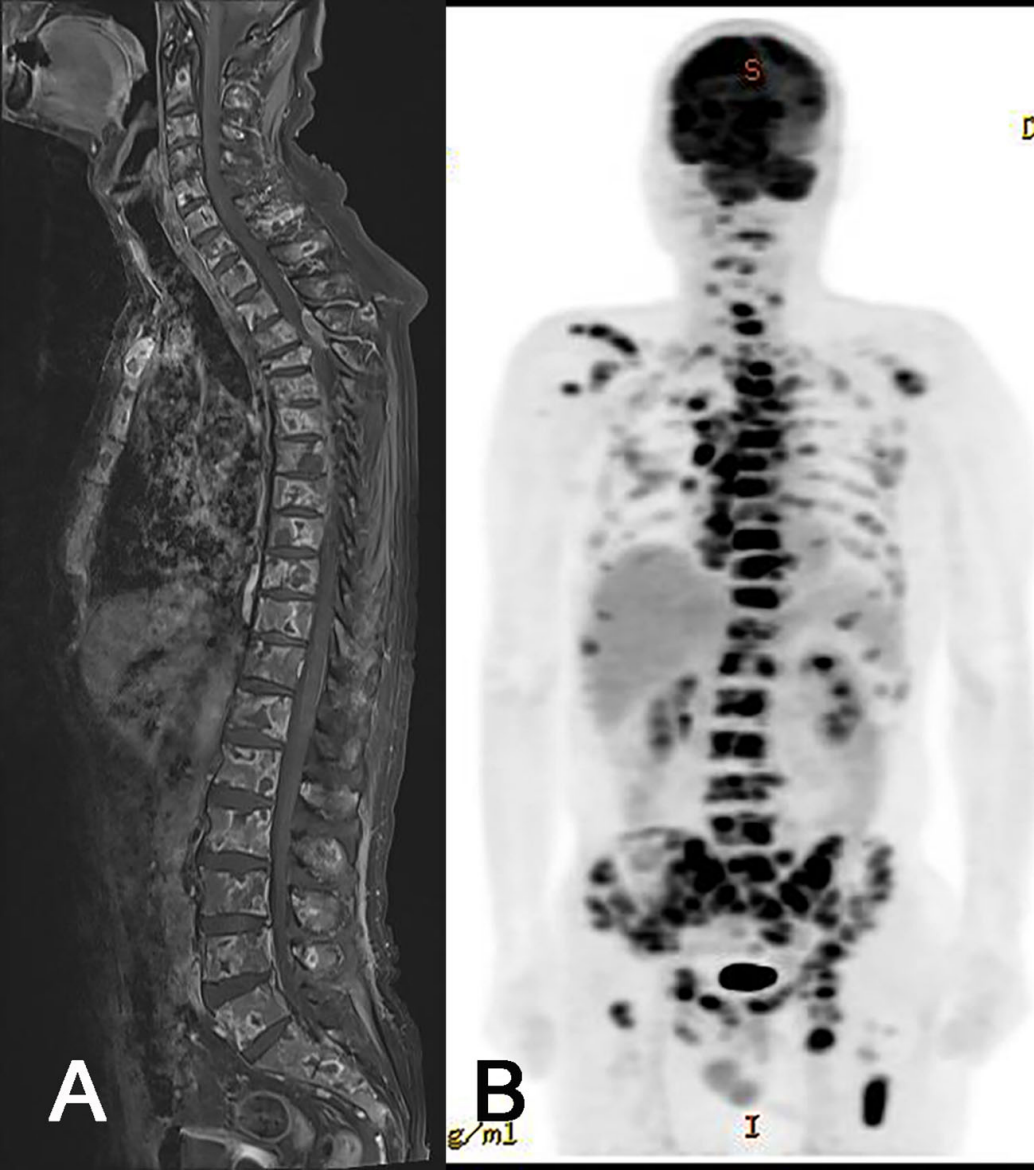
Radiologic images of extraneural metastasis (ENM). Whole spine MRI (A) and PET-CT (B) showing extensive ENM to multiple bones and lymph nodes.
